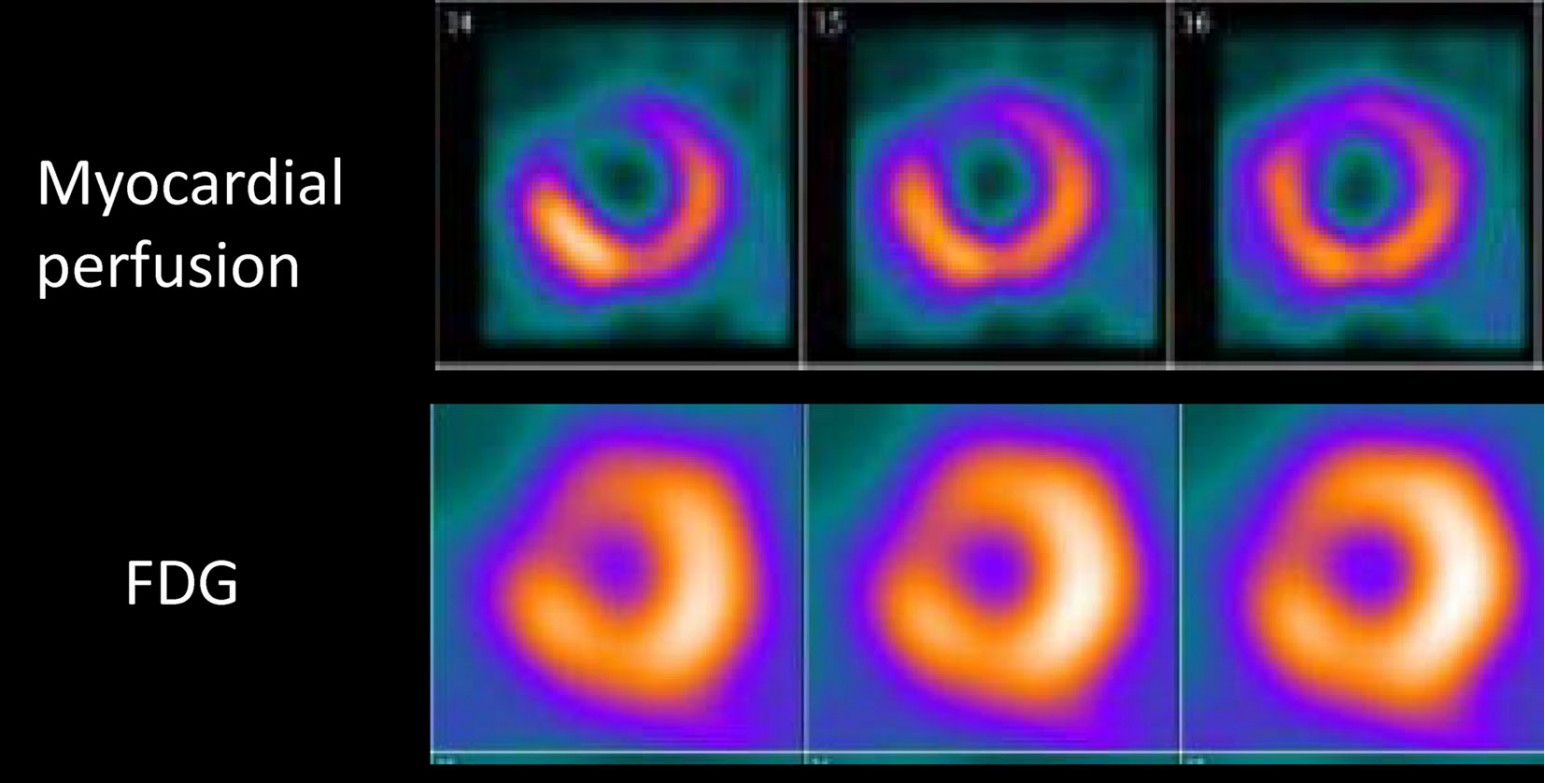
Example of a 76-year-old patient with previous anterior myocardial infarction and 2-vessel coronary artery disease on invasive coronary angiography (significant long lesion on the proximal left anterior descending coronary artery and dominant circumflex coronary artery with a significant lesion proximal). Left ventricular ejection fraction was 27%. Selected short-axis views of myocardial perfusion ^99^technetium tetrofosmin SPECT images show a perfusion defect in the anteroseptal wall. On fluorine^18^-deoxyglucose (FDG) SPECT images segments, uptake of radiopharmaceutical in the anteroseptal wall is visualized indicating perfusion-metabolic mismatch, pattern of myocardial viability
